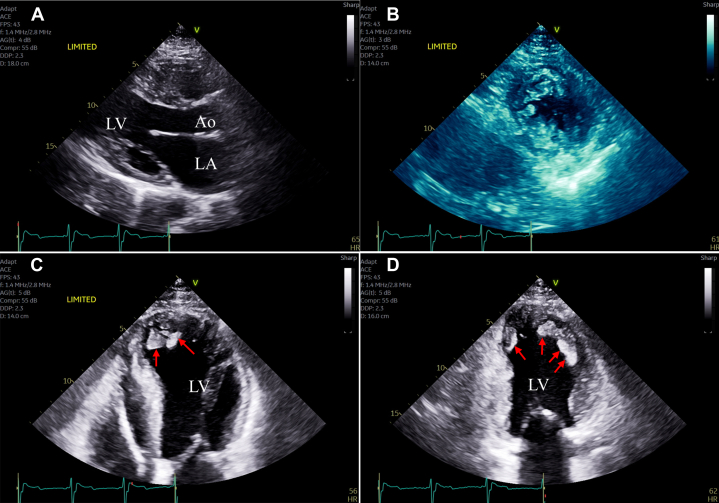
Complicated Features of Noncompaction With a More Extensive LV Thrombus The patient's echocardiographic images at age 28. The parasternal long-axis view in diastole with further LV cavity dilation and regression of hypertrophy (A). The parasternal short-axis view in systole with noncompaction (B). Apical 4- (C) and 2-chamber (D) views with an extensive thrombus (red arrows). Ao = aorta; LA = left atrium; LV = left ventricle.
